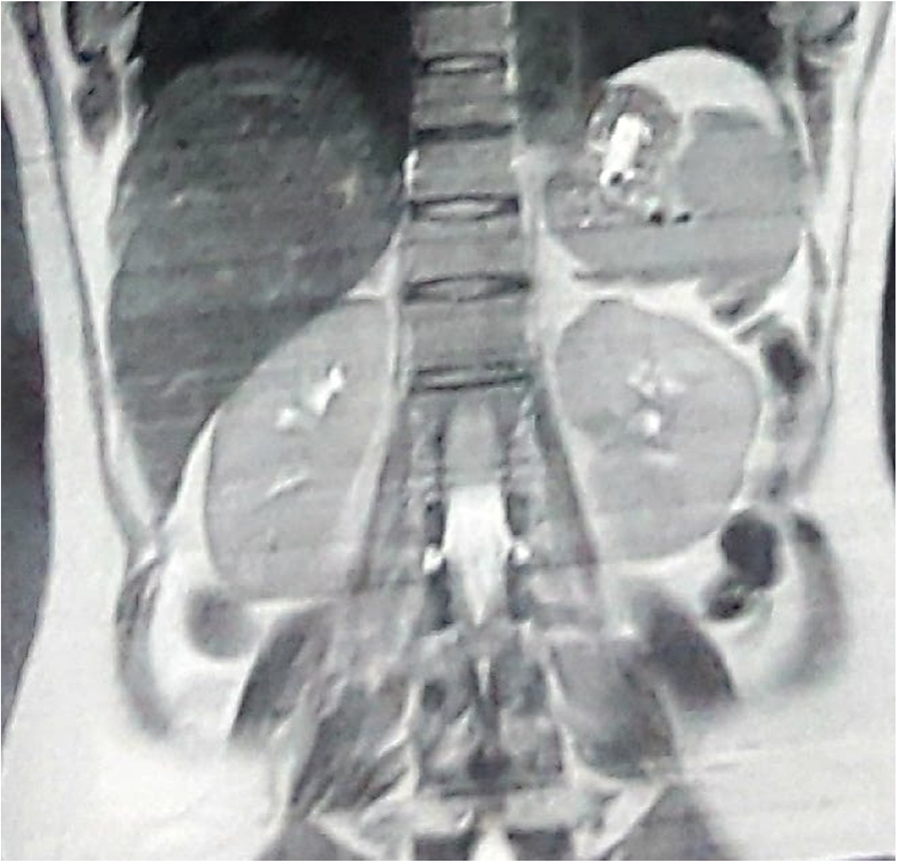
Magnetic resonance imaging showing a well-defined 100 × 95 mm heterogeneous mass in the left adrenal region above the kidney
